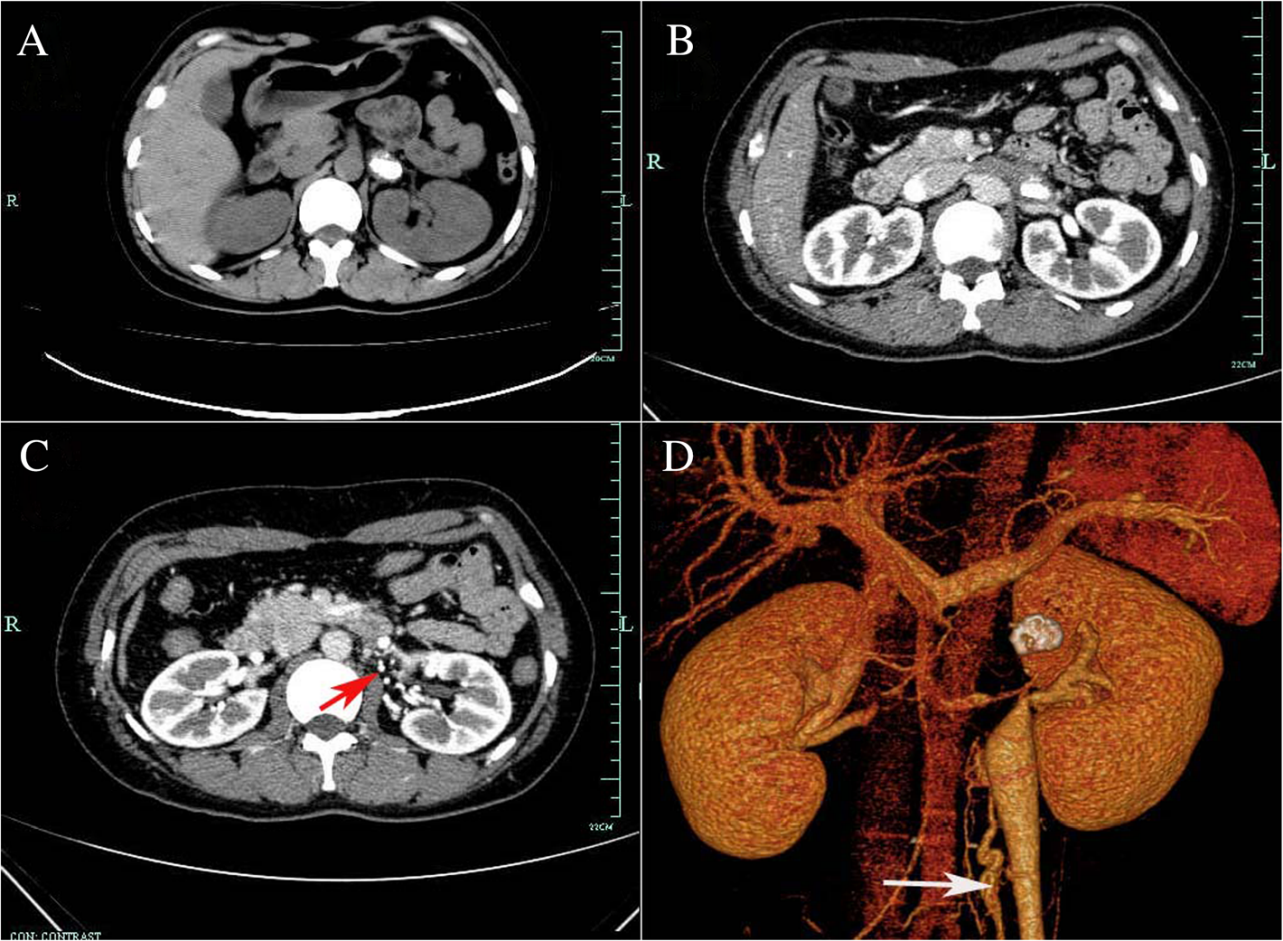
The images from the computed tomography (CT). a The noncontrast CT demonstrated left ureter calculus. b The contrast enhanced CT indicated a hyperdense mass in the left renal vein. c The contrast enhanced CT indicated peripheral veins (*red arrow*) around the left renal hilum. d Three-dimensional CT clearly displayed the calcified RVT with varicose ovary vein (*white arrow*)
